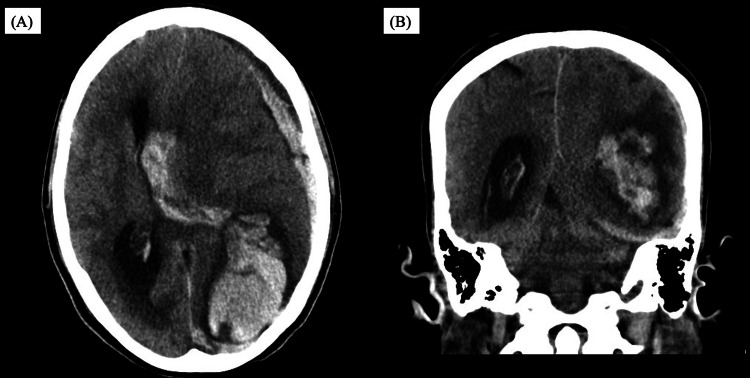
Head computed tomography on day 10 showing intracranial hemorrhage (A) Axial view showing a large left occipital intracerebral hemorrhage with intraventricular extension. (B) Coronal view confirming extensive intracerebral hemorrhage with ventricular involvement.
